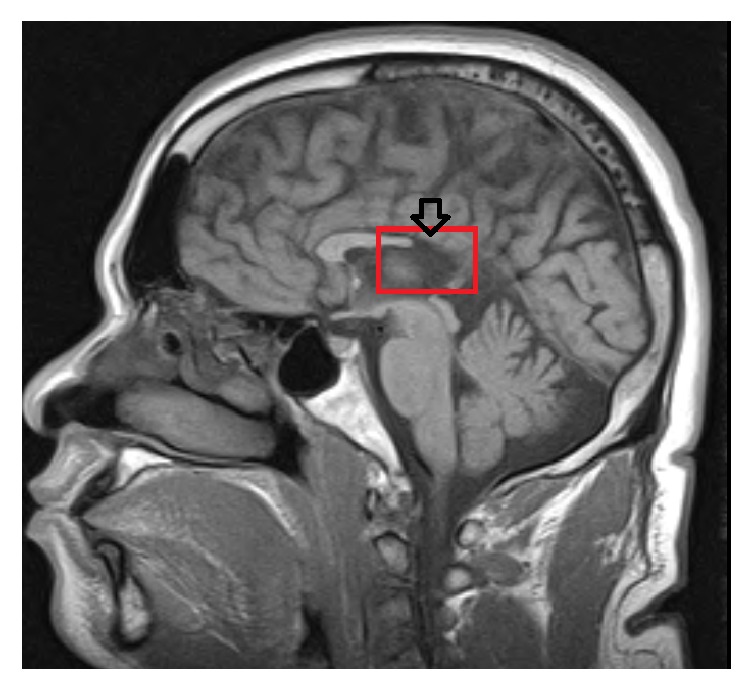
MRI demonstrating partial agenesis of the corpus callosum with the absence of the posterior body and the splenium.
